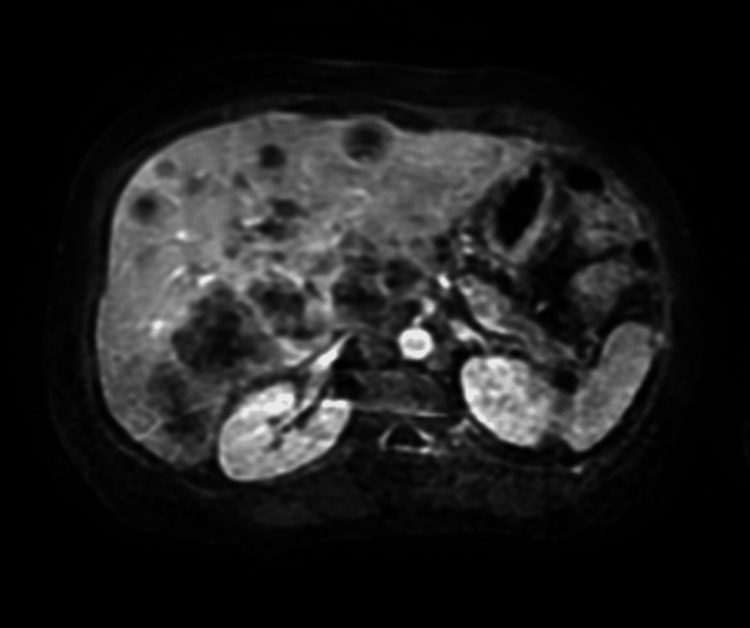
CT scan showing multifocal liver lesions, retroperitoneal lymphadenopathy, omental caking, mild pelvic ascites, and two small pulmonary nodules (2 mm and 3 mm).
